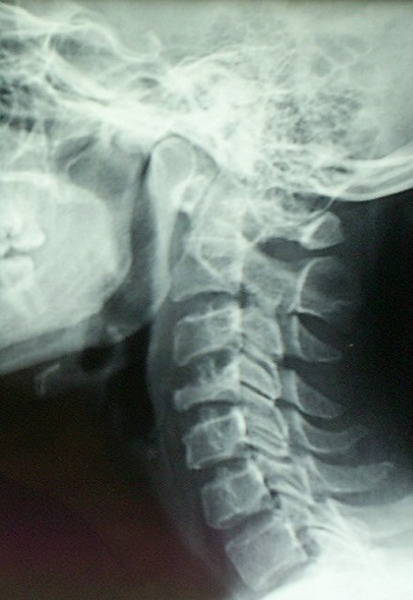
standard radiography showed osteolysis with compression of the vertebral body of C4
